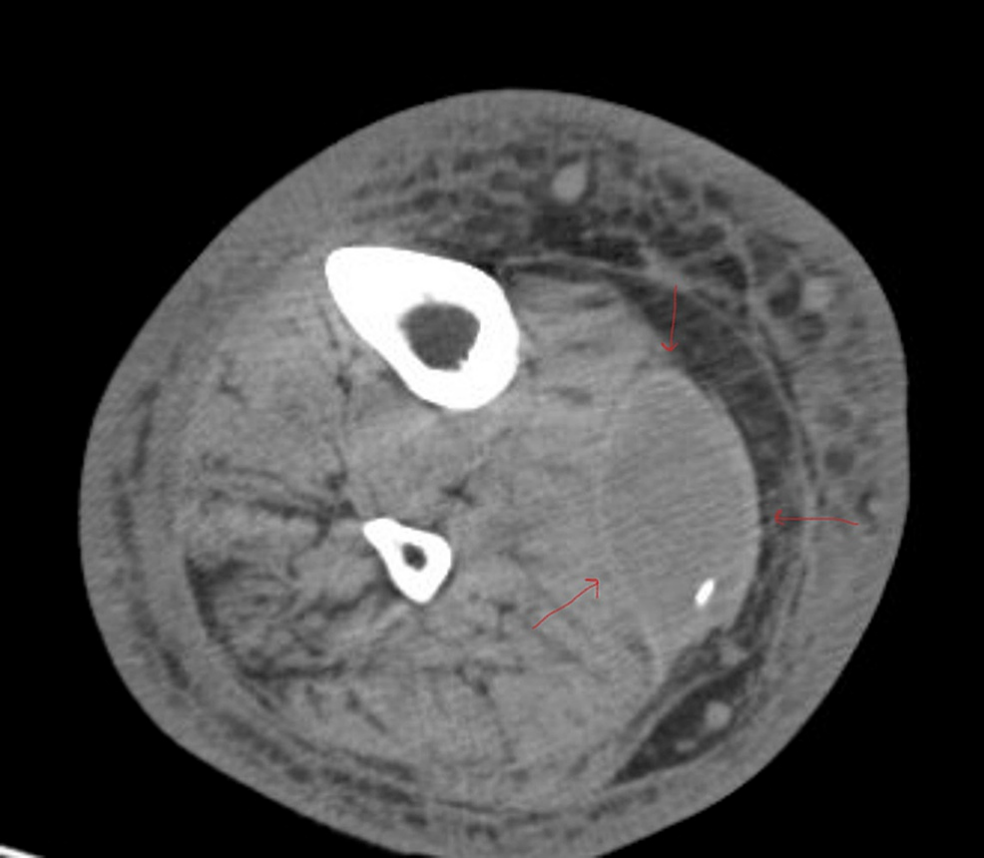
CT scan showing the intramuscular hematoma CT: computed tomography
